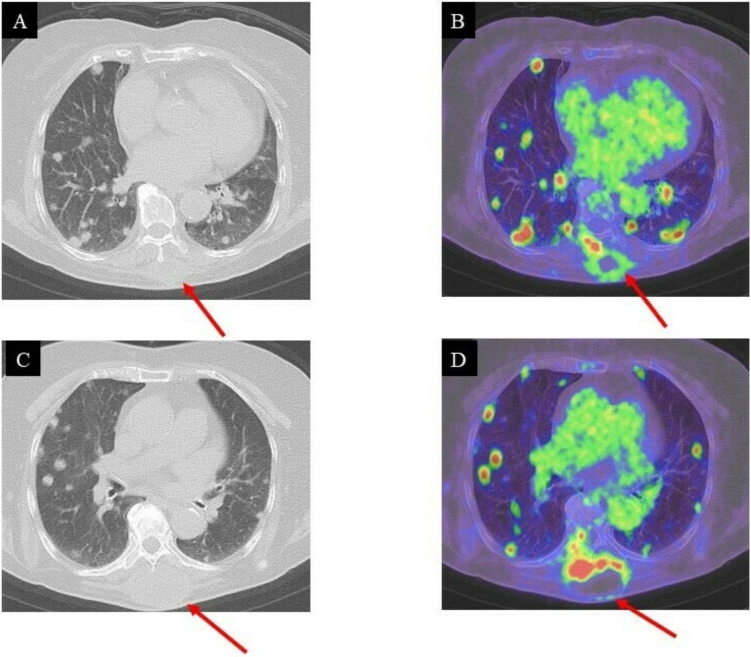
CT and PET/CT images of the lung three months after surgical resection: local recurrence and multiple pulmonary metastases; (A,B), (C,D): high FDG uptake in the intrinsic back musculature (SUV max = 14.96) and bilateral lung lesions (SUV max = 7.15–16.22), respectively.
